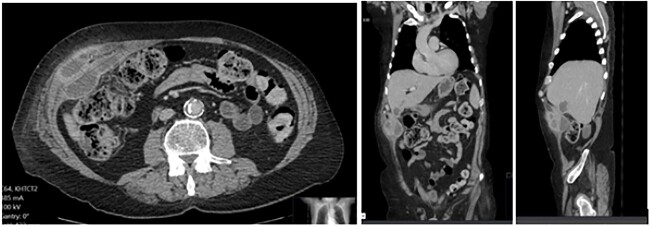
CT abdomen with portal venous contrast showing gallbladder collection extending to lateral abdominal wall.
